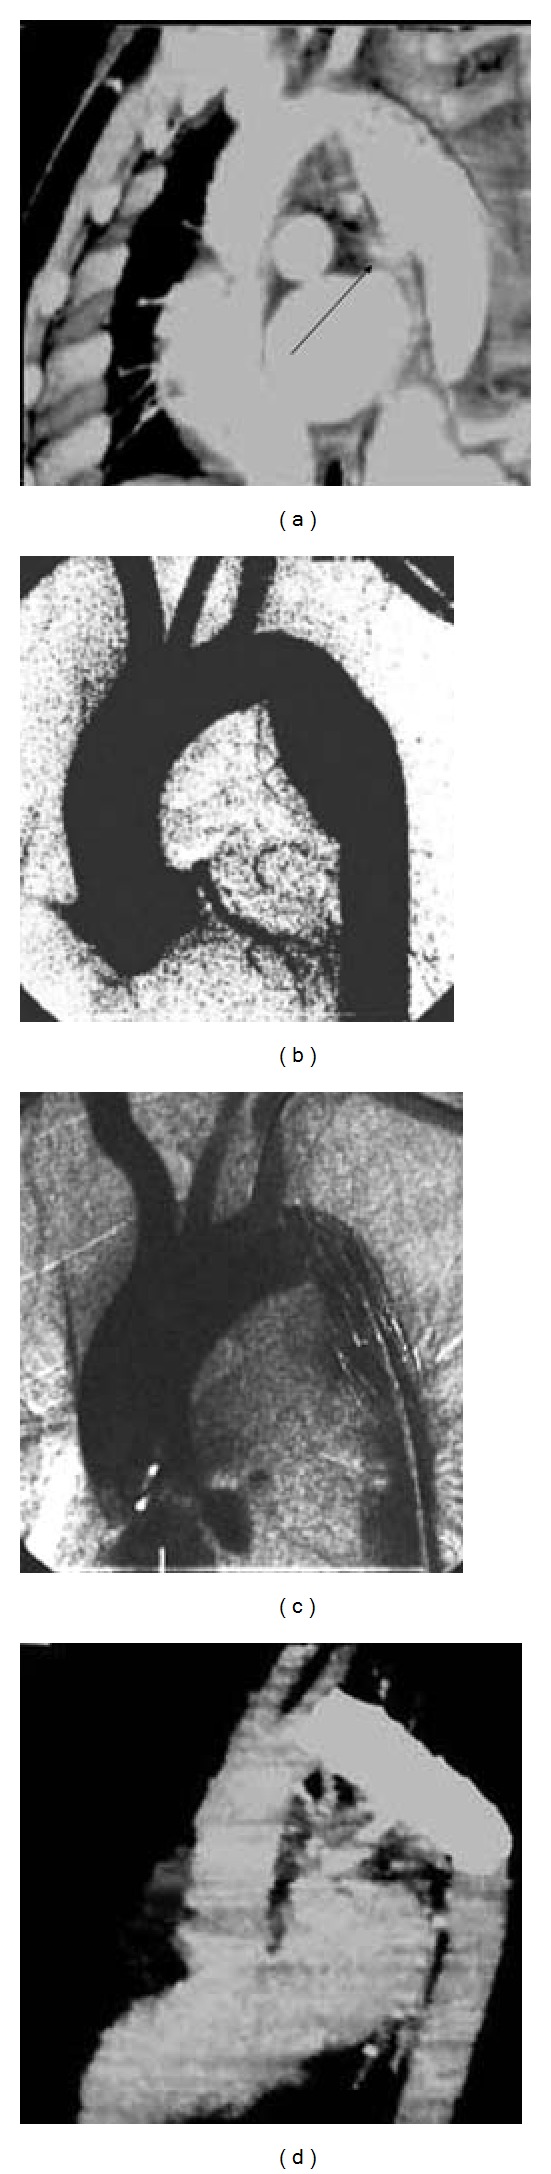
(a) MRI showing a spindle-shaped aneurysm at the beginning of the descending aorta with a suspected rupture in its inner wall (arrow); (b) DSA showing a fusiform aneurysm at the top of the descending aorta; (c) the lesion is properly treated by an endograft; (d) follow-up MRI showing a well-deployed endograft two years after deployment.
